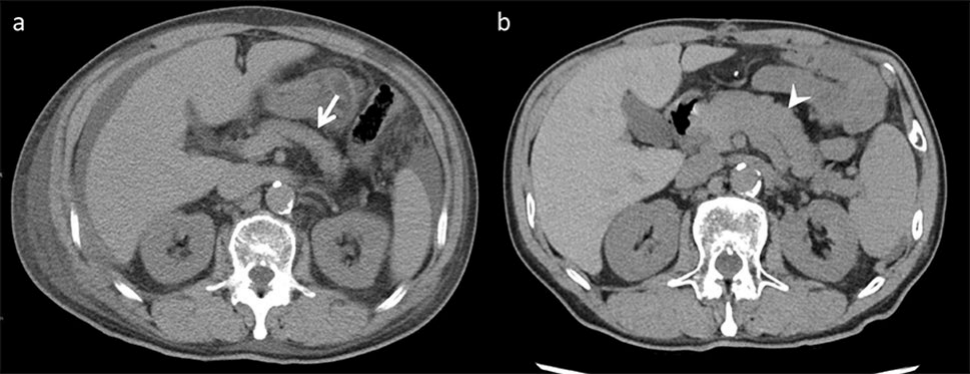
Pancreatic enlargement in an asymptomatic 70-year-old man with pulmonary arterial hypertension (PAH), who was receiving epoprostenol therapy for PAH. a Unenhanced computed tomography (CT) demonstrates the normal pancreas size before epoprostenol therapy (arrow). b Pancreatic enlargement is observed on unenhanced CT performed four months after the epoprostenol initiation (arrowhead). On imaging, acute pancreatitis is a differential; however, the absence of retroperitoneal fat stranding is a key to distinguishment
